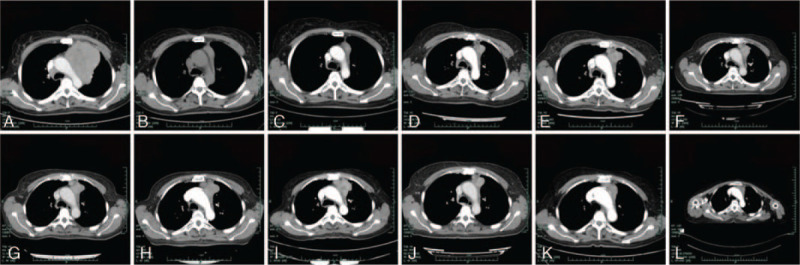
Chest CT scan showing the mass on the left upper lobe. A. Nov. 14 2014, B. May 5 2015, C. Oct. 28 2015, D. Dec. 20 2015, E. Sep. 28 2016, F. Jan. 23 2017, G. Mar. 11 2017, H. June 8 2017, I. Nov. 13 2017, J. Sep. 7 2018, K. Dec. 4 2018, L. Apr. 6 2019.
